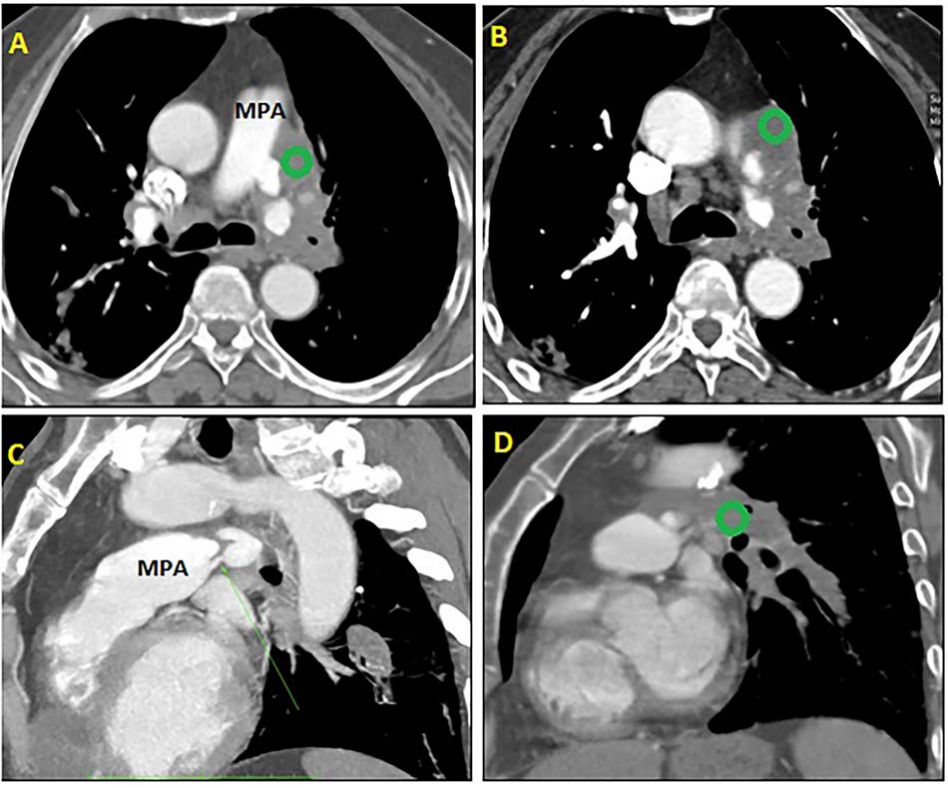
CT scan: axial (A + B) and sagittal (C + D) views showing a multivesicular hydatic cyst (green circle) class CE2 WHO, with severe reduction of the flow and partial occlusion of the left pulmonary artery. MPA: main pulmonary artery.
